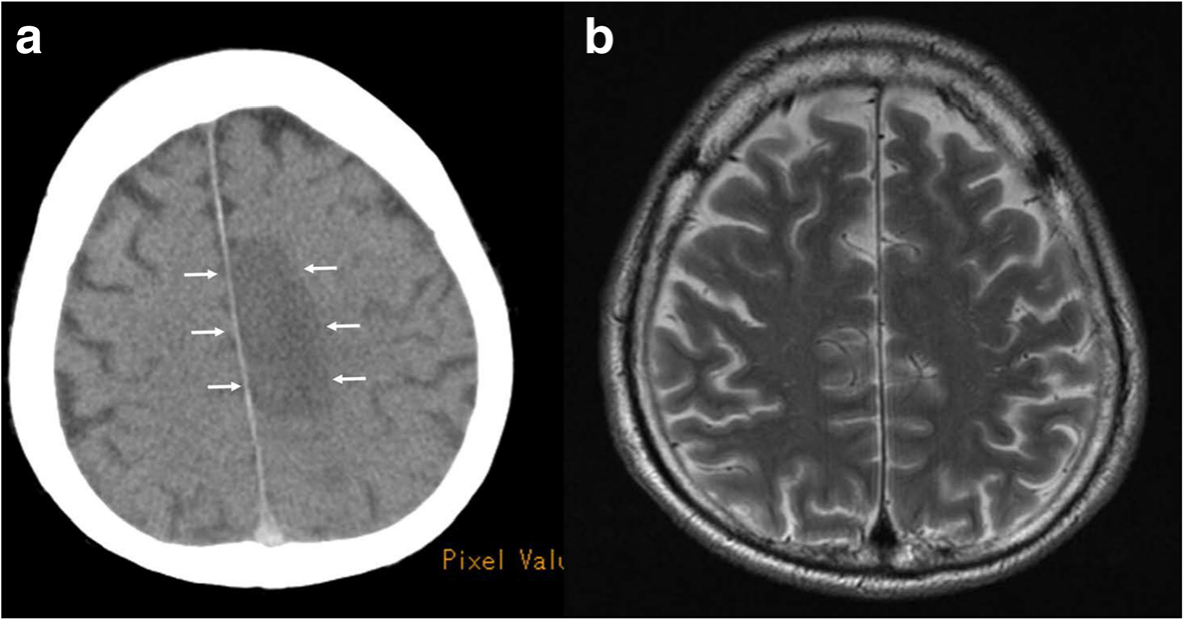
The postmortem CT and antemortem MRI (Case 3). a Brain postmortem CT image 7 h after death. The left parietal lobe has an LDA that was diagnosed as cerebral infarction (*arrows*). b Antemortem MRI, T2-weighted showing no cerebral infarction. The brain MRI was taken 22 days before death
